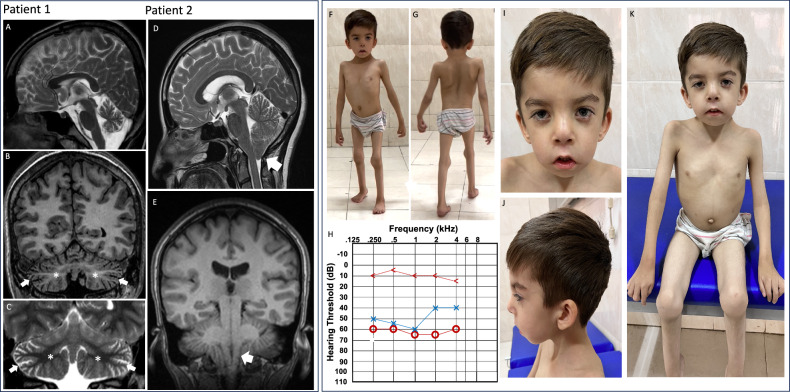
Clinical features of patients. Left panel: A–C Sagittal and coronal T2 weighted imaging (WI), and coronal T1-WI brain MRI imagines from proband 1, showing selective atrophy of the cerebellum including the vermis and cerebellar hemispheres (arrow, B, C) and abnormal signal changes affecting the dentate nuclei (asterisks, B, C). D, E Sagittal T2-WI and coronal T1-WI brain MRI imagines from proband 2 showing pointed cerebellar tonsils slightly displaced caudally resulting effacement of the CSF columns through the craniocervical junction (borderline Chiari type 1 deformity). Right panel: F, G Stills extracted from a walking video depicting proband 1’s gait. H Pure-tone audiogram for the proband 1, showing elevated air conduction thresholds in dB hearing level for the right (red circles) and left ears (blue crosses). Bone conduction thresholds for the right ear are indicated by ‘<‘. I–K Clinical photographs of the proband 1.
